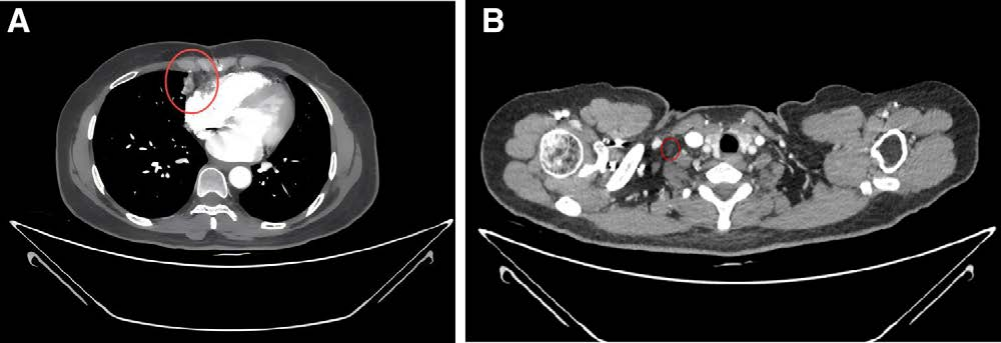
Chest CT follow-up on March 24, 2021. (A) Further reduction in the right lung lesion; (B) further reduction in the right supraclavicular lymph node.
